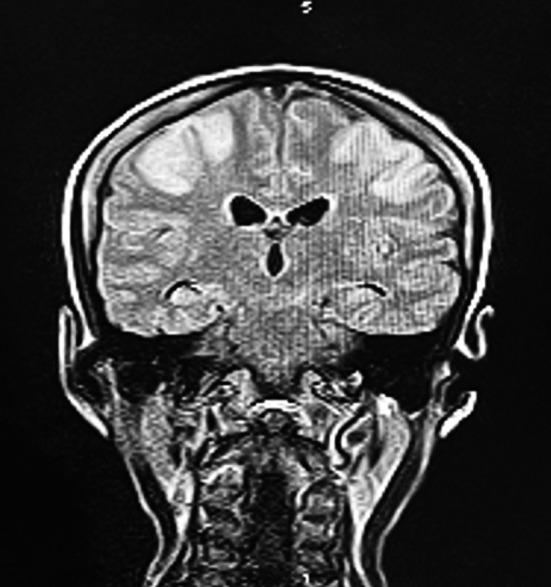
MRI brain showing hyperintense signal in bilateral parietal region. (Picture courtesy Dr. Muhammad Farid, dept. of Radiology, SIUT).
